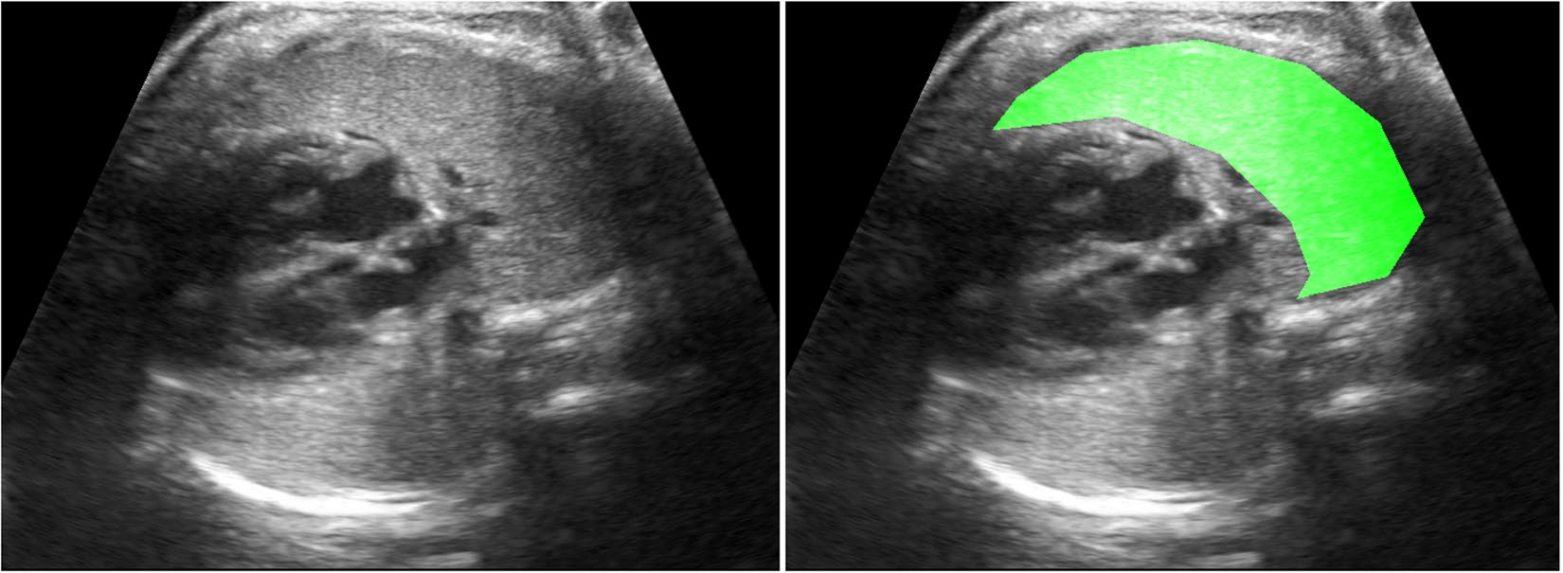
Example fetal lung ultrasound image and ROI marking the entire proximal lung.
